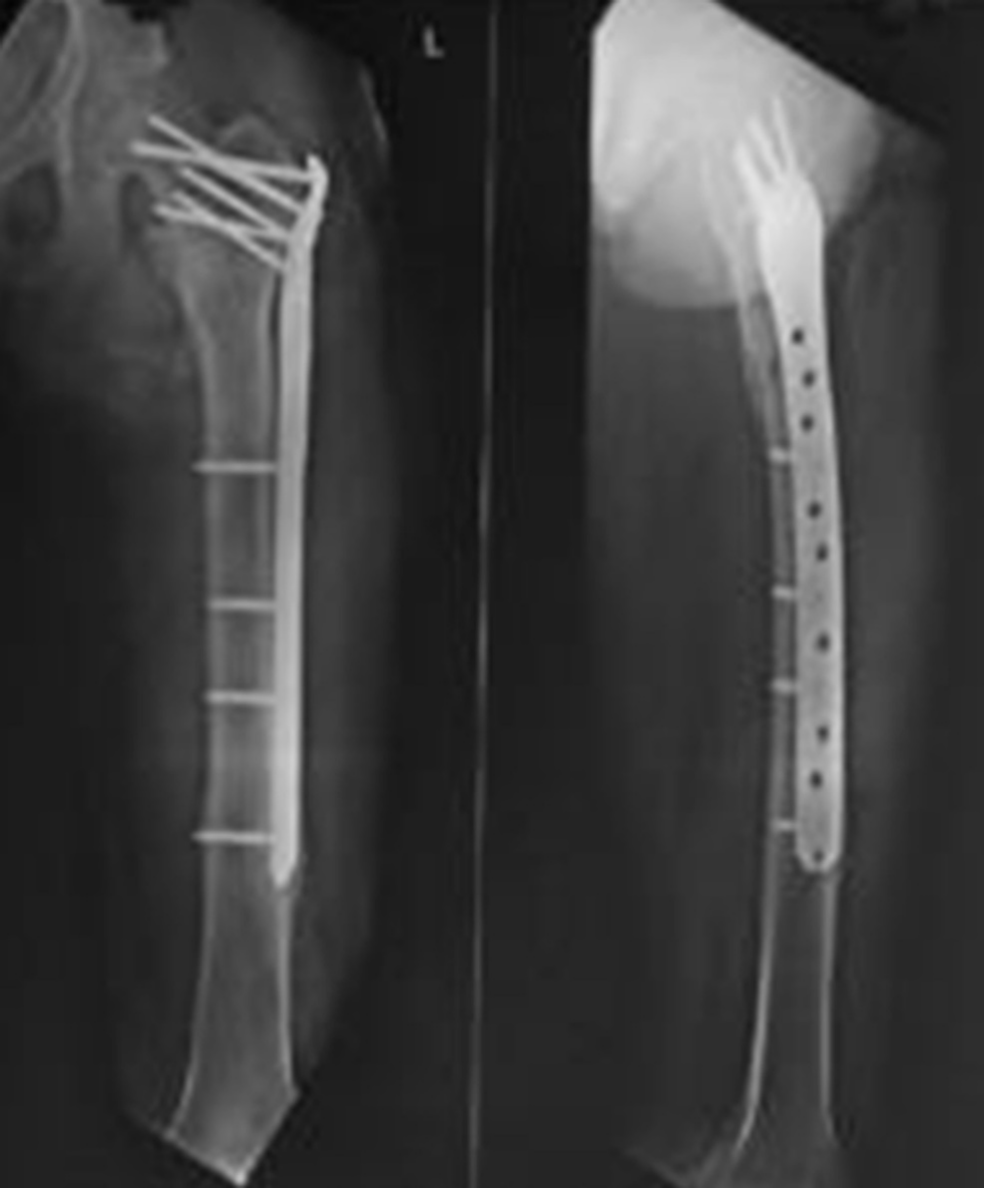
Antero-posterior and lateral radiographs at 12 months of follow up
